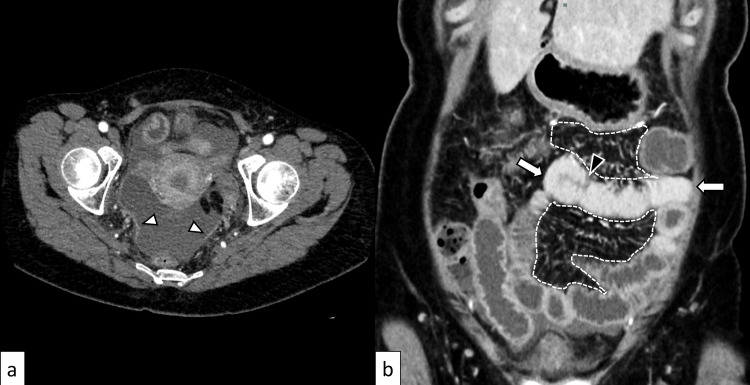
Contrast-enhanced abdominal computed tomography Peritoneal thickening with contrast enhancement is observed predominantly in the pelvis (a, white arrowheads). The small bowel demonstrates apparently uniform wall thickening resulting from obliteration of spaces between Kerckring's folds due to mucosal and villous swelling (b, arrows). Serosal swelling is also present (b, black arrowhead), along with dilation of adjacent mesenteric vessels (b, dotted lines).
